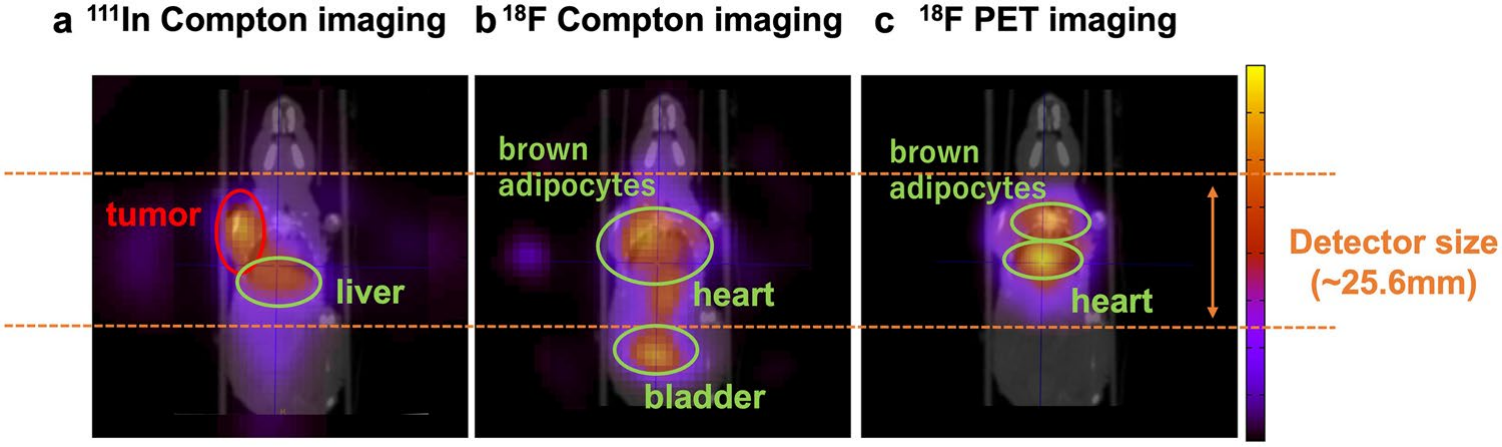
Simultaneous ^111^In and ^18^F in vivo imaging results. (a) A reconstructed image of the ^111^In antibody obtained by Compton imaging. The CT image and the reconstructed image are superimposed. The location of the tumor and the liver are visualized. (b) A reconstructed image of ^18^F-FDG obtained by Compton imaging. The location of the bladder is strongly visualized. (c) A reconstructed image of ^18^F-FDG obtained by PET imaging. The accumulation in the field of view (FOV), which is determined by the detector size, is visualized.
